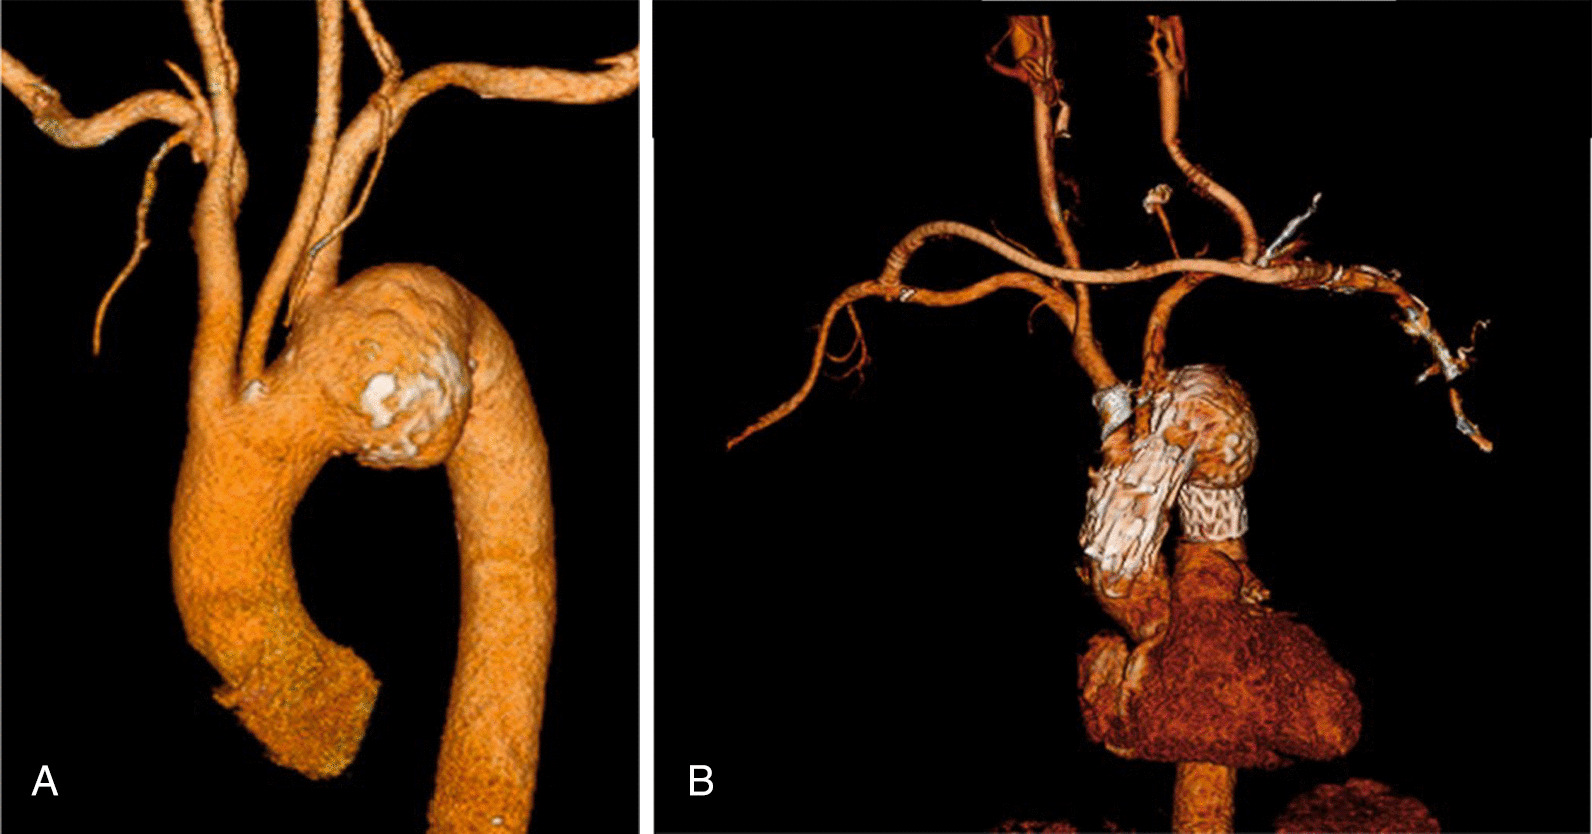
A Acute aortic arch angulation and a distal arch aneurysm involving the left subclavian artery are also shown on the preoperative computed tomography scan. B Using 8 mm heparin-bonded expanded polytetrafluoroethylene grafts, an extra thoracic bypass from the right subclavian artery to the left subclavian artery and to the left common carotid artery was accomplished
